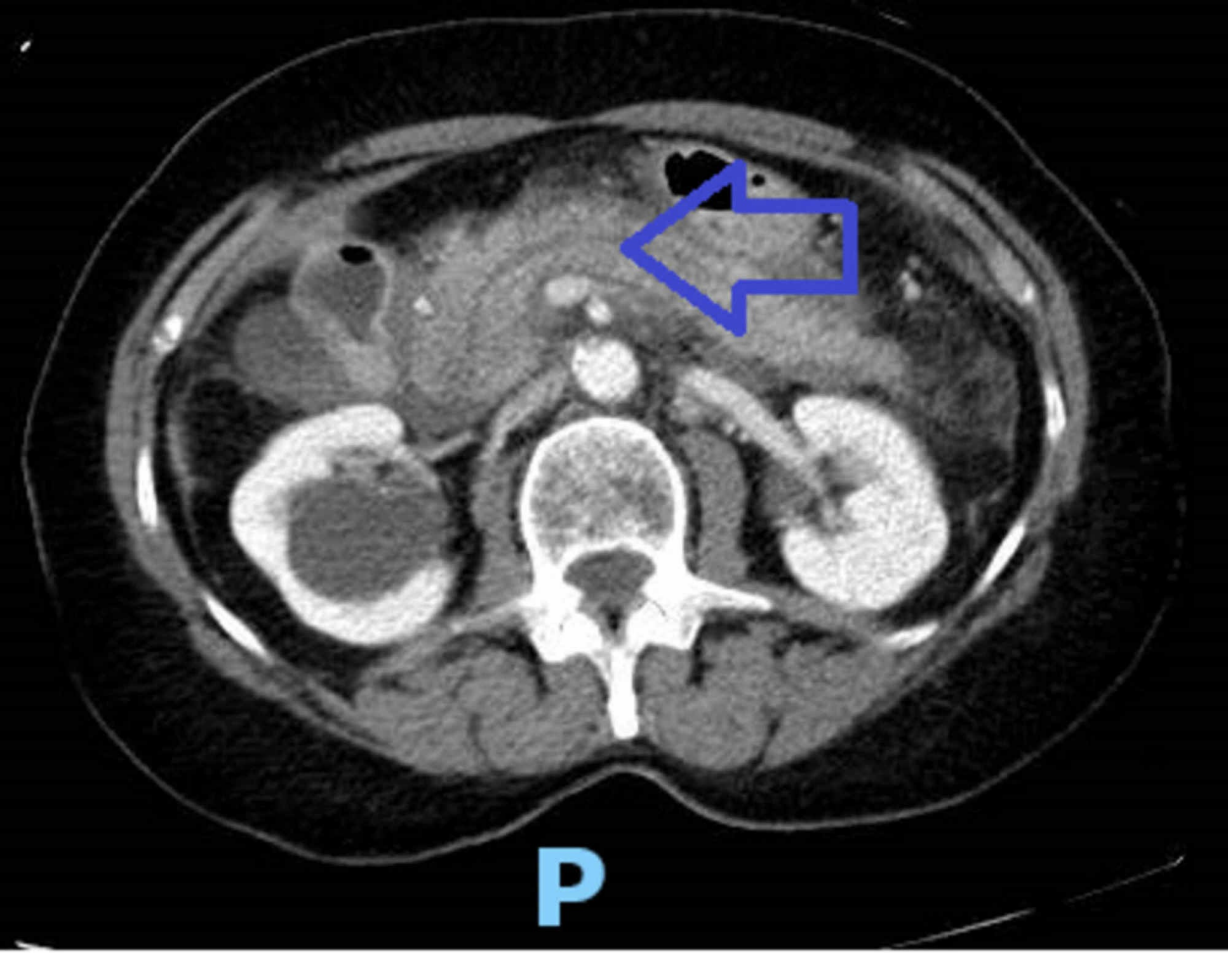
CT abdomen The arrowhead points at the inflamed pancreas.
